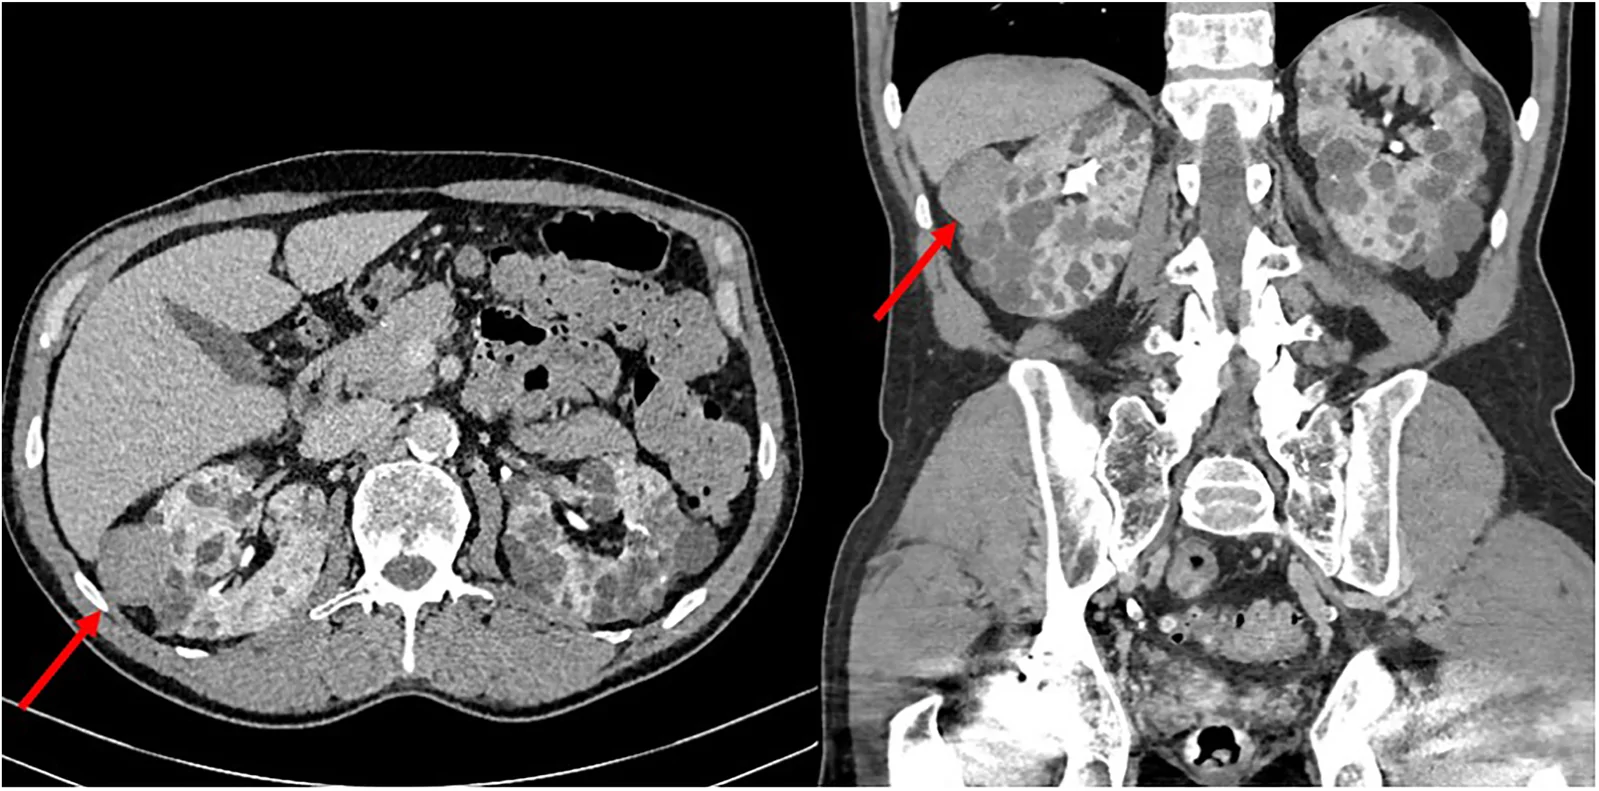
Case 1. Axial and coronal computed tomography images of the 4cm exophytic right renal mass in a patient with autosomal dominant polycystic kidney disease
